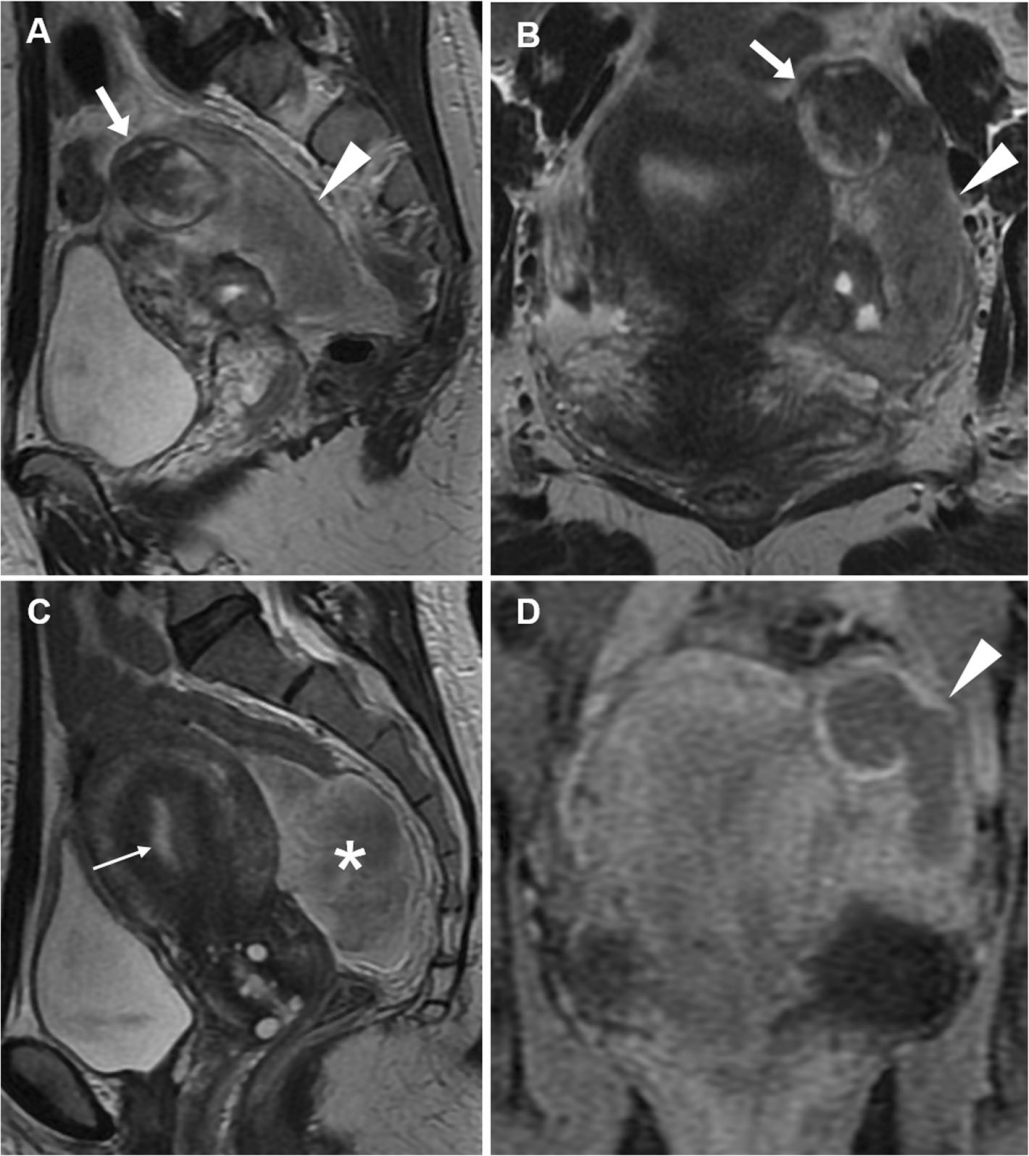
MRI of a ruptured first-trimester ectopic pregnancy in a 44-year-old woman with acute abdominal pain and elevated β-hCG levels. Sagittal (a) and oblique-coronal (b) T2-weighted images show a tubular left adnexal mass (arrowheads) containing a gestational sac in the isthmus of the fallopian tube (arrows). On oblique-coronal fat-suppressed T1-weighted image (d), the distended fallopian tube displays a thick hyperintense wall (arrow) consistent with haematosalpinx. Additionally, sagittal T2-weighted image (c) demonstrates a predominantly low-signal haemorrhagic fluid collection (asterisk) in the pouch of Douglas. Note the empty endometrial cavity (thin arrow)
